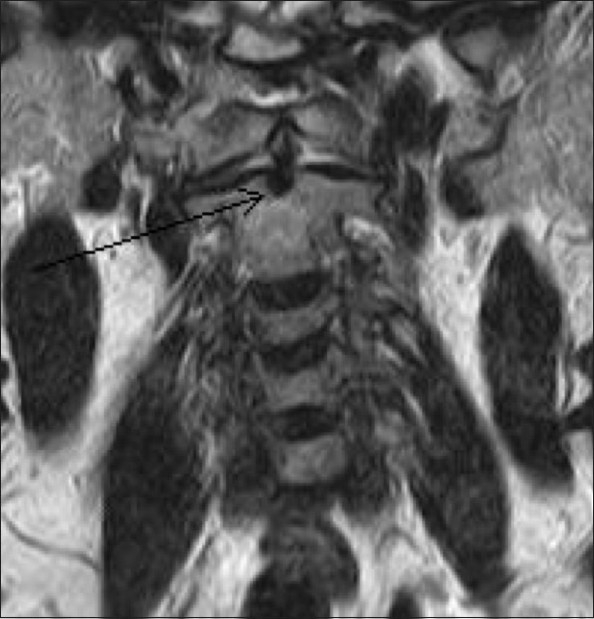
Coronal T2W MRI image shows a well-defined depression (arrow) on the superior aspect of the body of C2 with non-visualization of the dens
